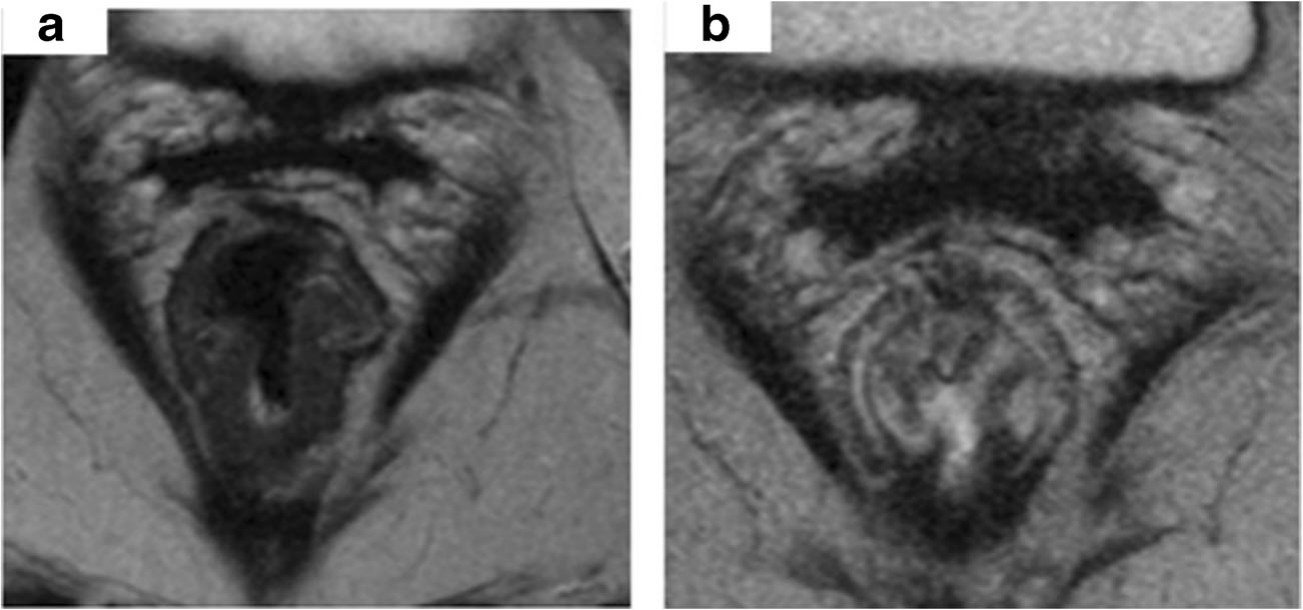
Low rectal tumour before treatment with full invasion of the muscularis at 4–8 o’clock position. The tumour borders the intersphincteric plane, therefore the CRM is involved at the level of the distal levators (a). Post CRT MRI demonstrates an area of low signal fibrosis at the site of the treated tumour, and no evidence of macroscopic residual intermediate signal suggestive of tumour—mrTRG2 (b)
